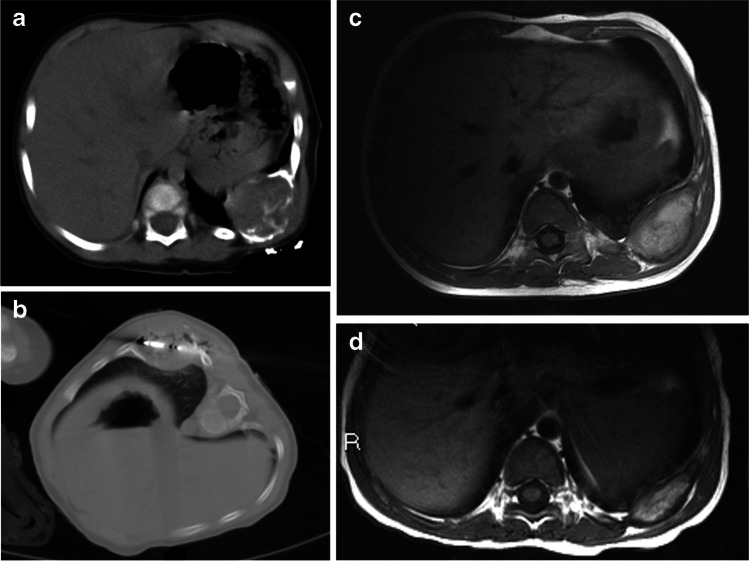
Mesenchymal hamartoma in an 11-month-old boy (patient 2) treated with microwave thermoablation. a, b He presented with a clinical mass of the 9th–10th left ribs, as confirmed on axial CT (with contrast agent) at diagnosis (a), and he underwent microwave thermoablation (axial CT, b). c, d Follow-up MRI. At first (1-month) follow-up, axial T1-weighted MRI (c) shows an inhomogeneous hyperintense lesion, which decreased in dimension on the 9-month follow-up axial T1-weighted MRI (d)
